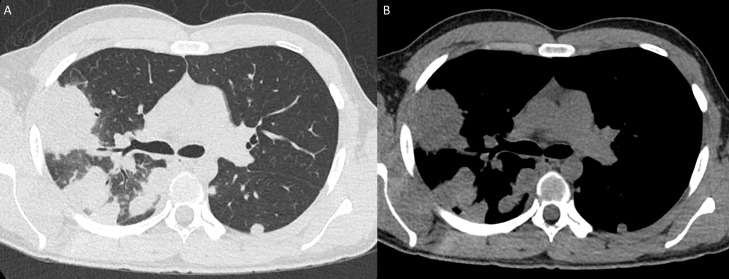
CT of the chest axial images, lung (A) and soft tissue (B) reconstruction algorithm, showed numerous (more than 30) bilateral pulmonary nodules/masses, ranging from 20-60 mm in diameter with associated peripheral halo. No pleural effusion or pneumothorax and patent central airway. Findings were favored to represent vasculitis.
